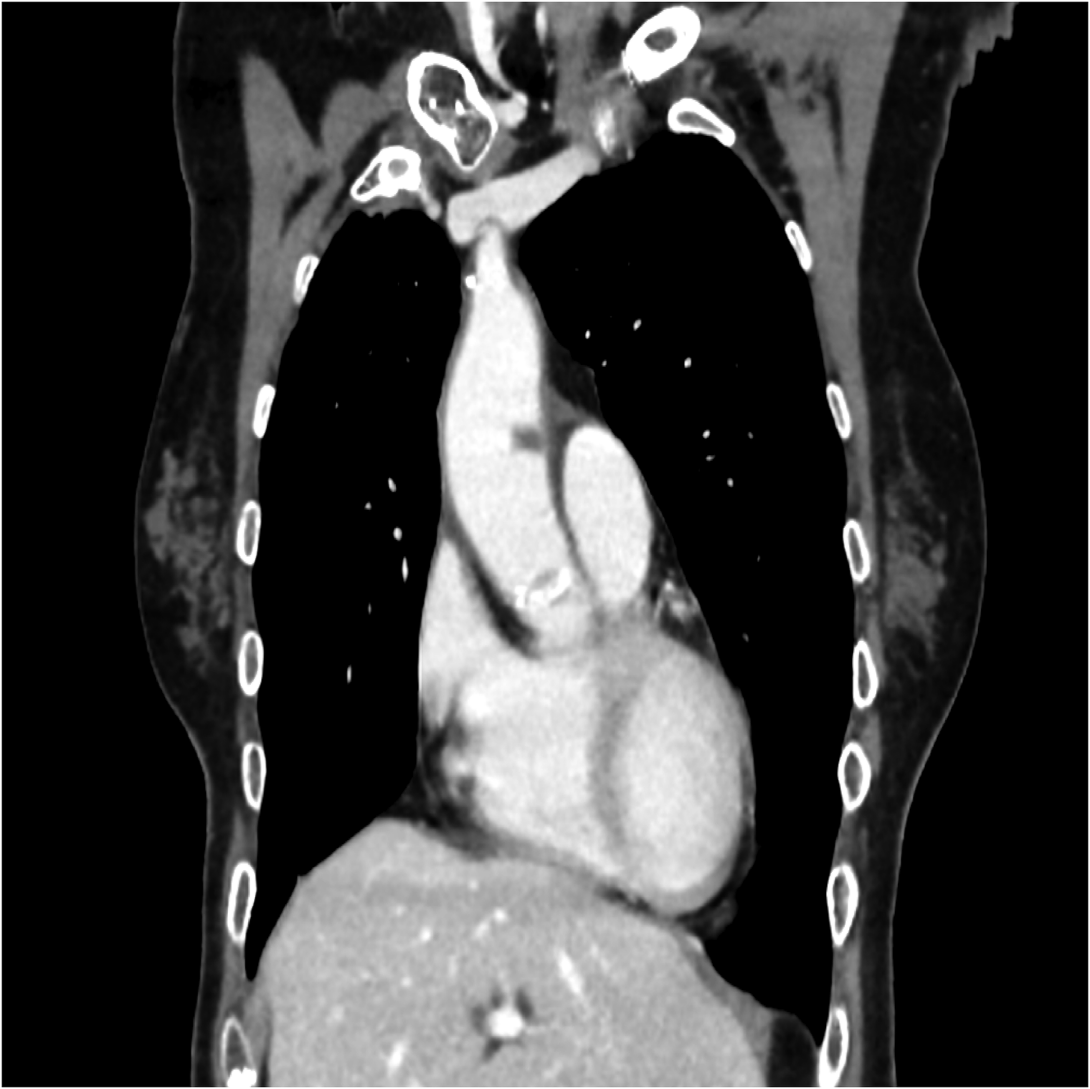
Coronal CT-scan plane showing the 8 mm thrombus in the ascending aorta.
